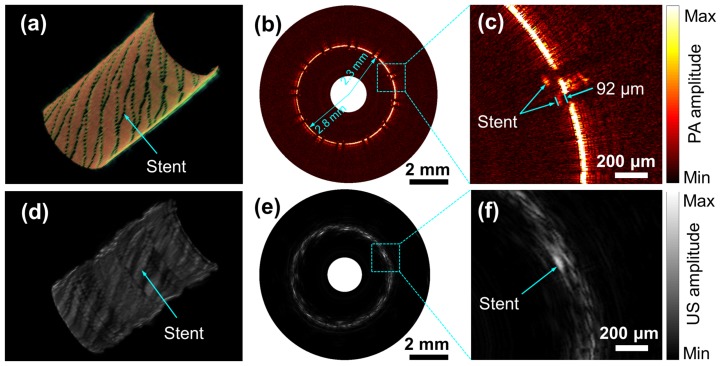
Photoacoustic and ultrasonic imaging with OR-PAT of a stent deployed in a plastic tube. Three-dimensional cut-away (a) photoacoustic and (d) ultrasonic images. Representative (b) photoacoustic and (e) ultrasonic B-scans. Enlarged photoacoustic (c) and ultrasonic images corresponding to the dash boxes in (b) and (e).
